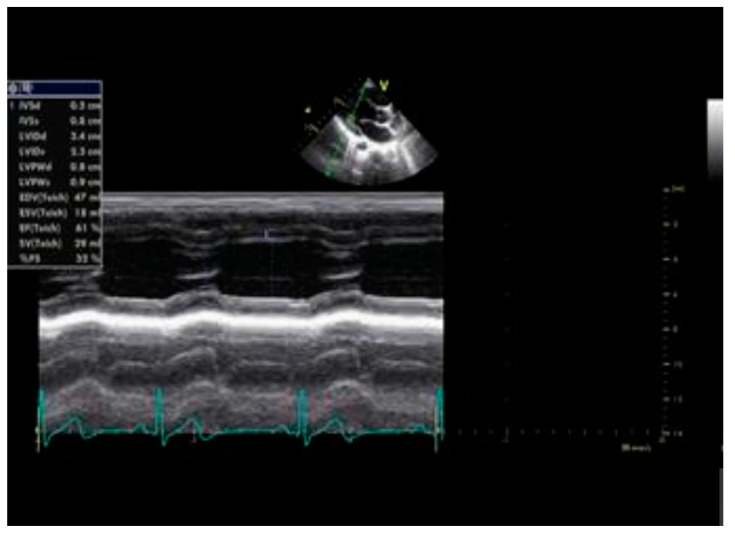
Shows M mode echocardiogram in a healthy child.
